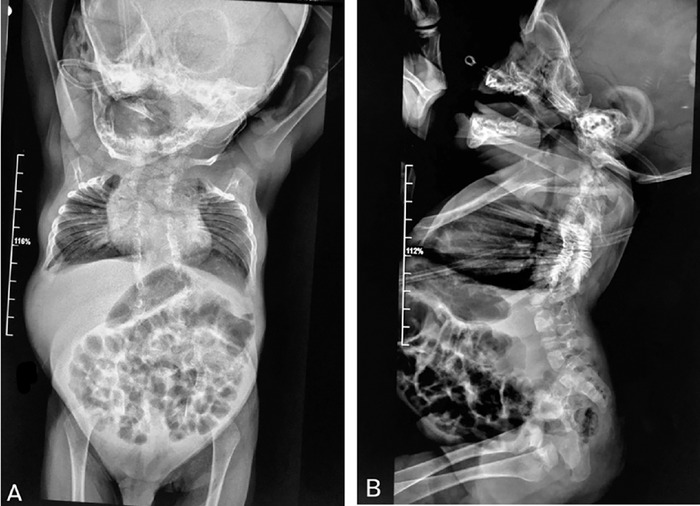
(A) Anteroposterior and (B) lateral radiographs of the thoracolumbar spine with kyphoscoliosis show abnormalities in the formation, segmentation, and curvature of the spine, predominantly at the thoracic level; abnormal closure of the posterior arches; and synostosis of the arches of the first and second ribs.
